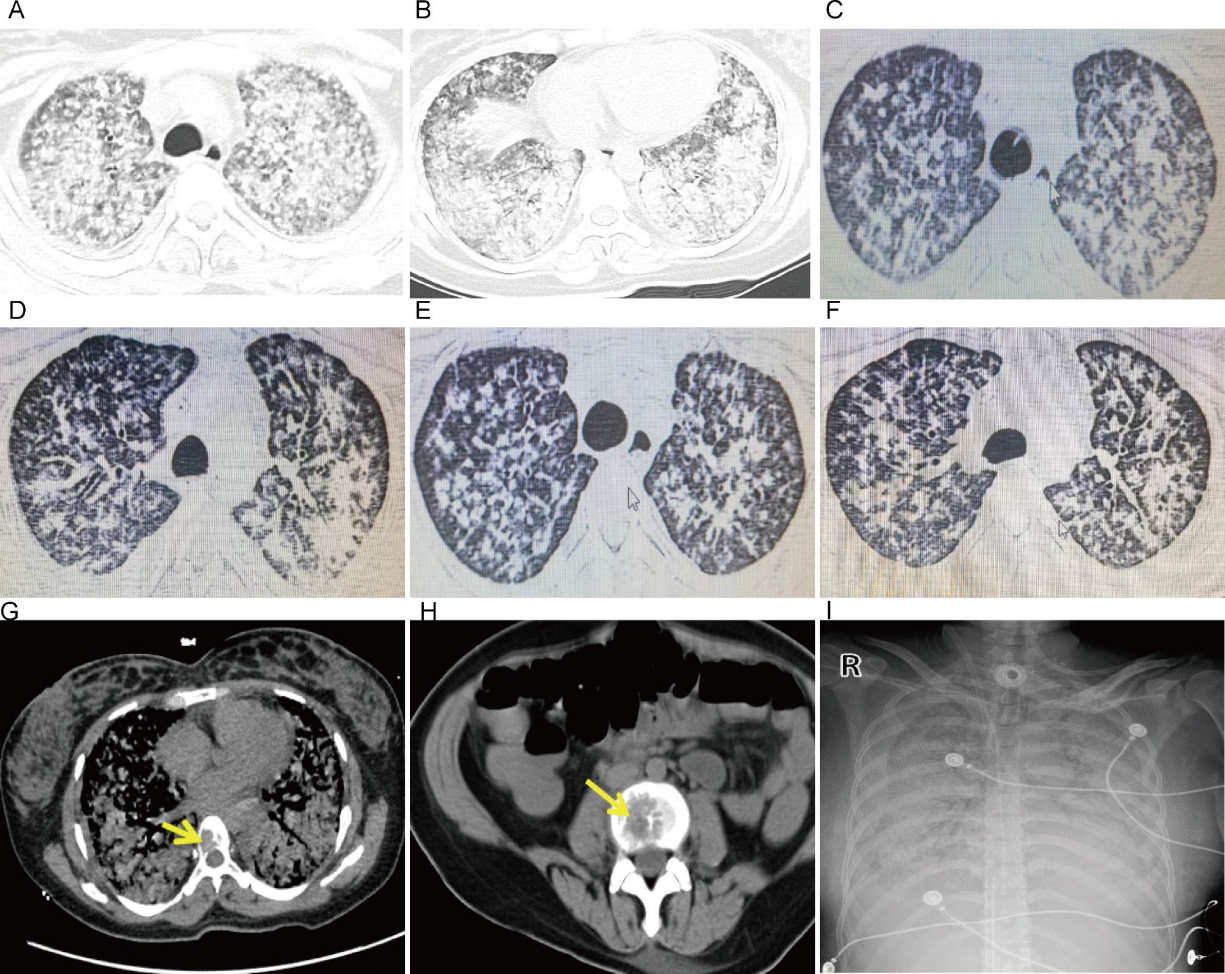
Changes in chest imaging during the patient’s hospitalization. (A and B) After delivery, the patient’s chest CT showed multiple exudative lesions in both lungs, multiple vertebral bone destruction in the thoracic vertebrae, and fractures in the 7th rib on the right and 2nd and 6th rib on the left. (C and D) On May 2, the reexamination of chest CT showed that multiple patchy high-density shadows were seen in both lungs, with blurred edges. (E and F) On May 9, re-examination of chest CT revealed fewer bilateral lung lesions compared to May 2nd. (G and H) The reexamination of spinal CT showed that multiple thoracic and lumbar vertebrae were damaged. (I) On May 19th, a bedside chest X-ray revealed diffuse exudative lesions in both lungs
